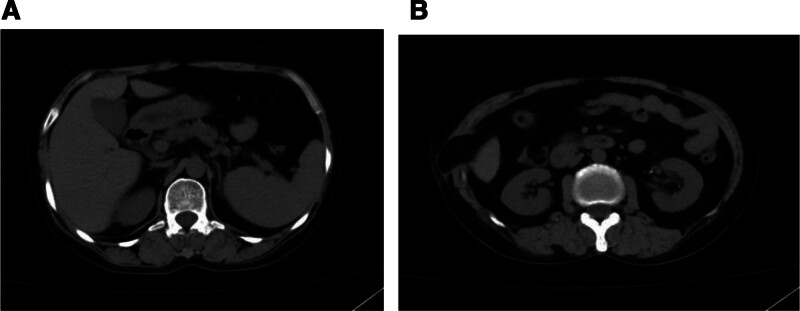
(A and B) Postoperative CT confirmed complete reduction of the hepatic parenchyma into the anterosuperior subphrenic space with restoration of normal anatomical relationships. CT = computed tomography.
